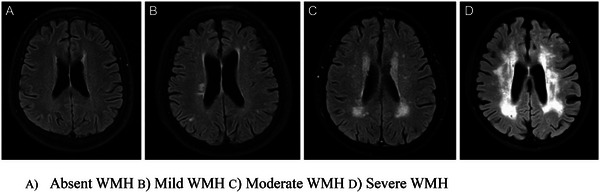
FLAIR images of different WMH severity. (A) Absent WMH, (B) mild WMH, (C) moderate WMH, and (D) severe WMH. WMH, white matter hyperintensities.
